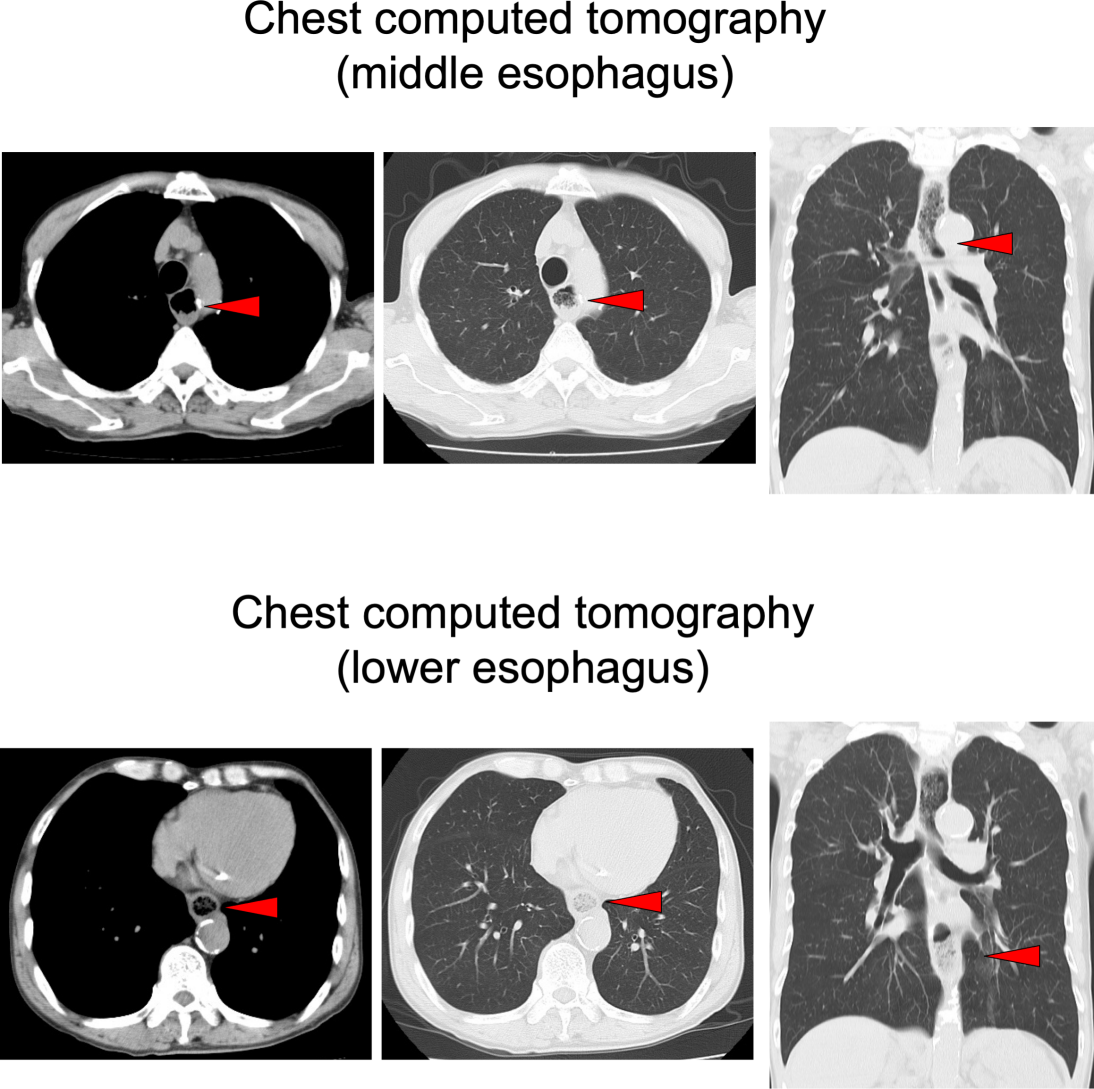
Chest computed tomography in the emergency room. A foreign body (red allow head) was detected in the middle esophagus (upper panels) and it was noted that the space was narrowed with the expansion of the upper lesion of the esophagus. Expansion of the esophagus was also observed in the lower esophagus (lower panels).
